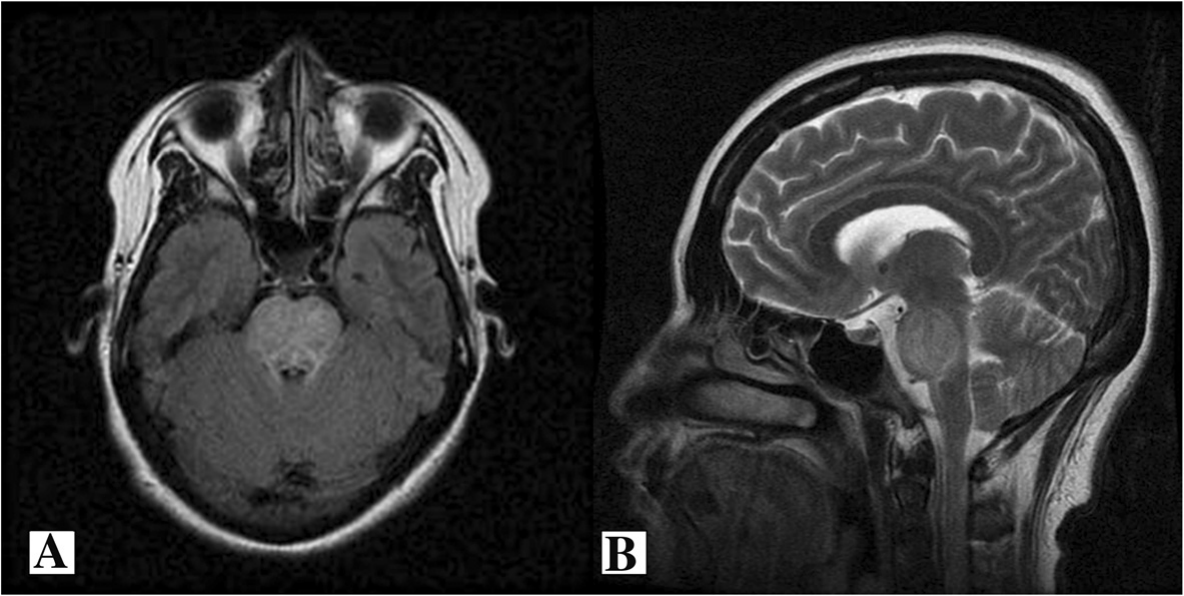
MRI of the brain revealed symmetric, high-intensity signal in the pons with sparing of the peripheral portion, suggesting central pontine myelinolysis (A, axial view and B, sagittal view).
